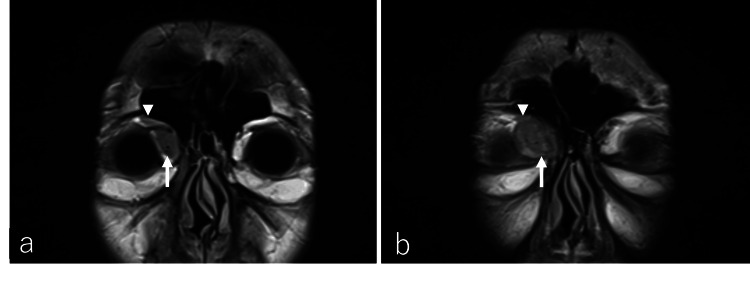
Coronal imaging findings of the right upper eyelid mass at initial diagnosis Coronal fluid-attenuated inversion recovery (FLAIR) images are shown in (a) and (b). The tumor was located in the medial aspect of the orbit (arrow). Its anterior portion extended to the upper eyelid and was in contact with the levator palpebrae superioris muscle (arrowhead).
